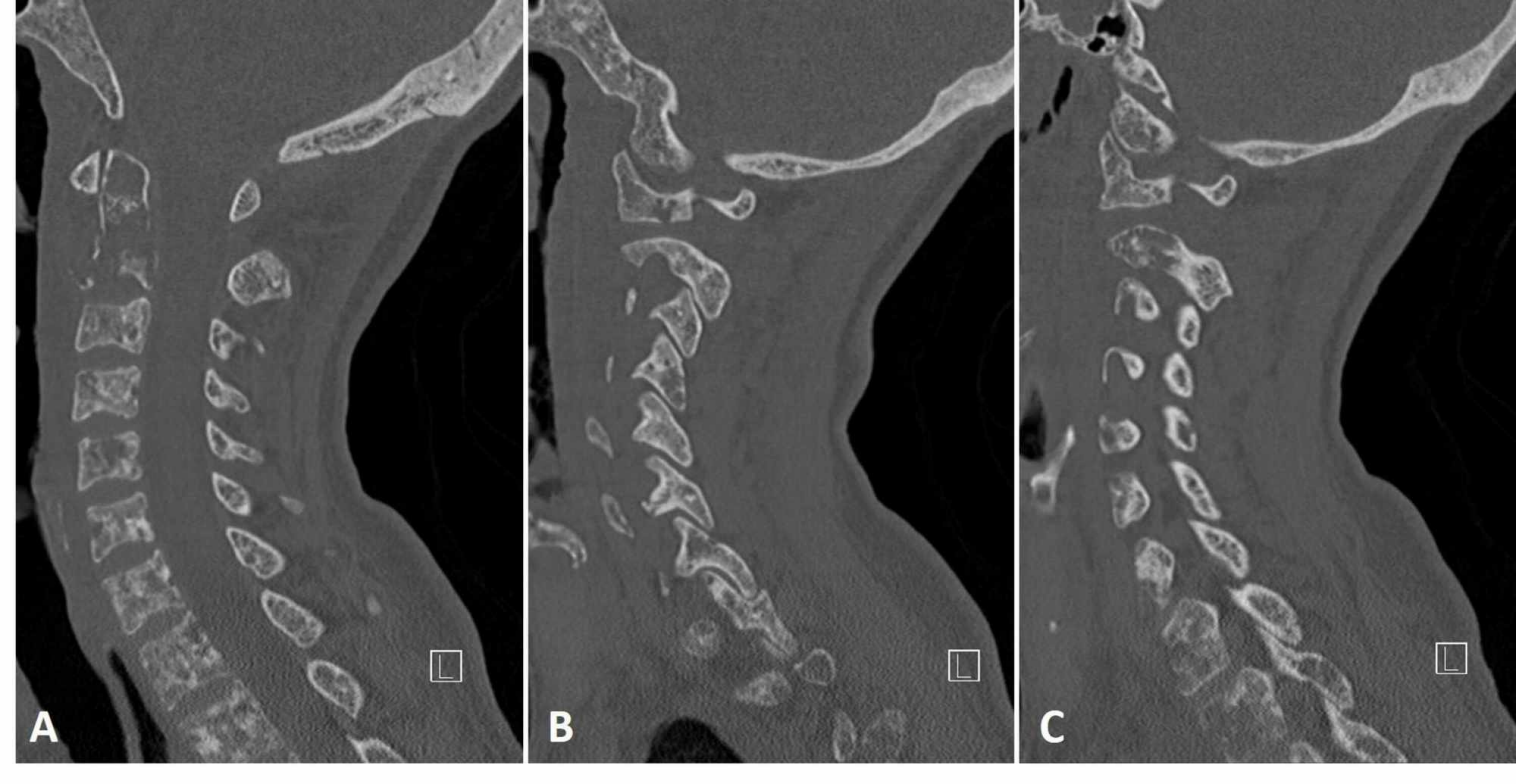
CT postapplication of crown halo traction CT postapplication of crown halo traction and subsequent vest placement showing realignment of the upper cervical spine (A: mid-sagittal) and reduction and realignment of the atlantoaxial joints bilaterally (B: left parasagittal and C: right parasagittal).
